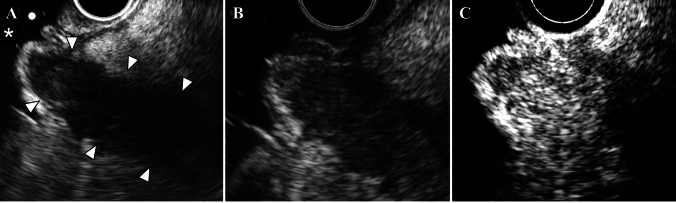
Endoscopic ultrasonography (EUS) findings of the duodenum lumen (dot), major papilla (asterisk), and the pancreatic head observed from the second portion of the duodenum. A, B EUS displayed a hypoechoic mass completely filling and conforming to the shape of the dilated main pancreatic duct (arrowhead). C On contrast-enhanced EUS, the mass showed a relatively homogeneous and delayed contrast effect
